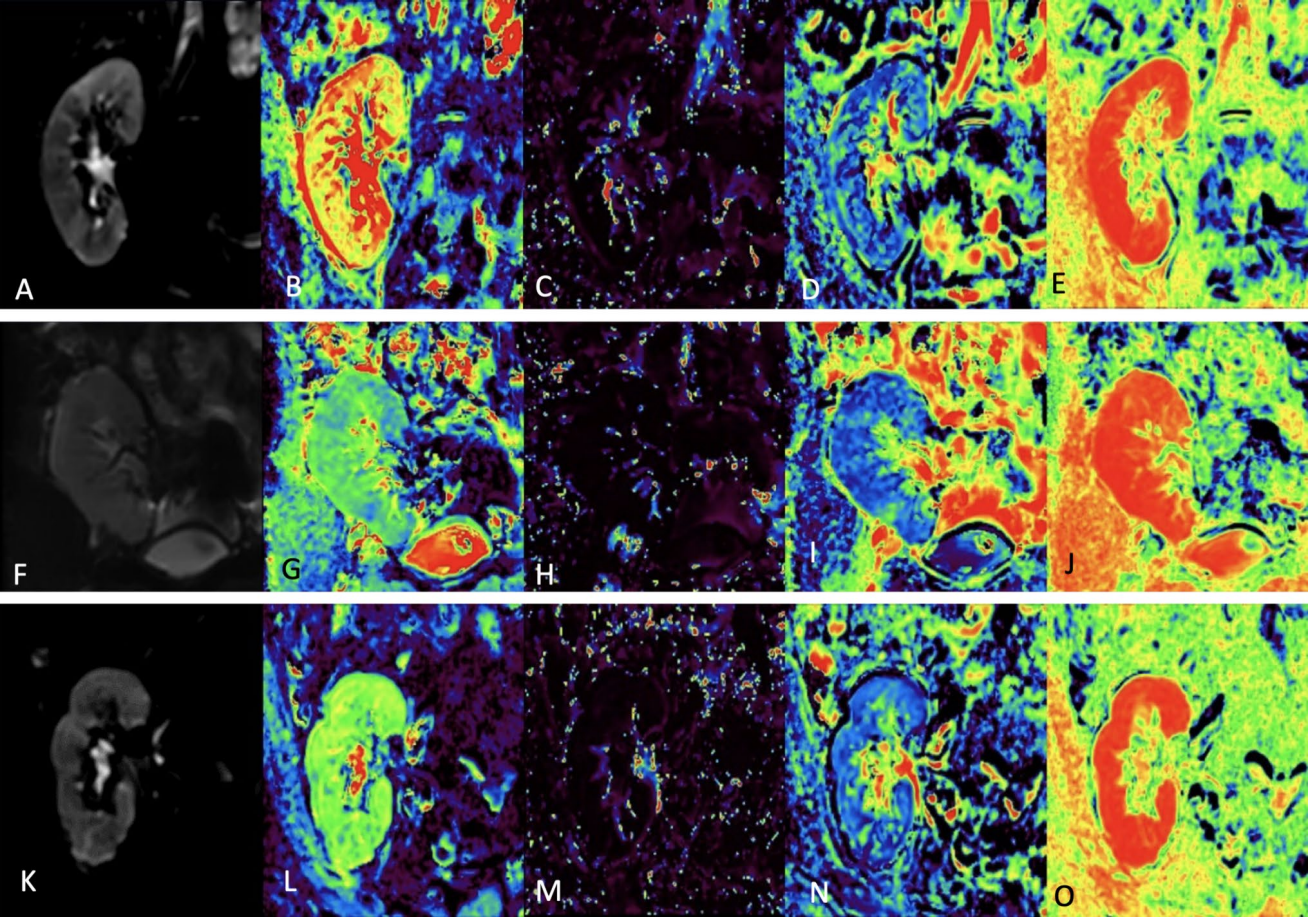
A–E was for a 21-year-old male with renal allograft transplantation 9 months ago with good renal allograft function; his scr level was 1.1 mg/dL with eGFR about 98 mL/min/1.73m^2^. IVIM-DWI MR parameter maps A b0 image, B D map, CD* map, D f map, and E Goodness of Fit. F–J was for a 25-year-old male with renal allograft transplantation 3 years ago presented with severe renal allograft impairment; his sCr level was 7.6 mg/dL from basal sCr level about 1.1 mg/dL with eGFR about 9 mL/min/1.73m^2^. He underwent renal allograft biopsy and was diagnosed as renal allograft rejection. IVIM-DWI MR parameter maps F b0 image, G D map, H D* map, I f map, and J Goodness of Fit. K–O was for a 53-year-old male with renal allograft transplantation 6 years ago presented with mild renal allograft impairment; his sCr level was 1.6 mg/dL from basal sCr level about 0.9 mg/dL with eGFR about 51 mL/min/1.73m^2^. He underwent renal allograft biopsy and was diagnosed as tubular necrosis on top of chronic renal allograft fibrosis. IVIM-DWI MR parameter maps K b0 image, L D map, M D* map, N f map, and O Goodness of Fit. *D* Diffusion coefficient, *D Pseudo-diffusion coefficient, *F* Perfusion fraction
